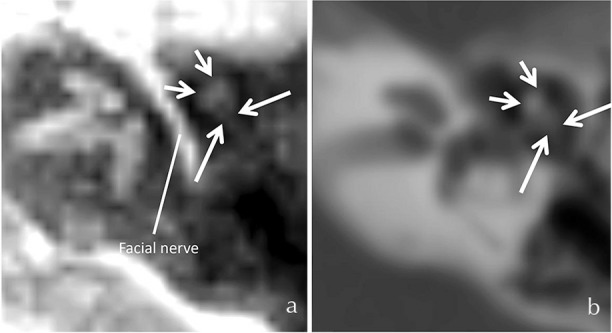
A 64-year-old woman. The head of the malleus (short arrows) and body of the incus (long arrows) in the left middle ear are visualized with a signal intensity between labyrinthine fluid and air on this PETRA image (a) which corresponds to the computed tomography image (b). Note that the facial nerve shows a higher signal than the labyrinthine fluid (a). PETRA, pointwise encoding time reduction with radial acquisition.
